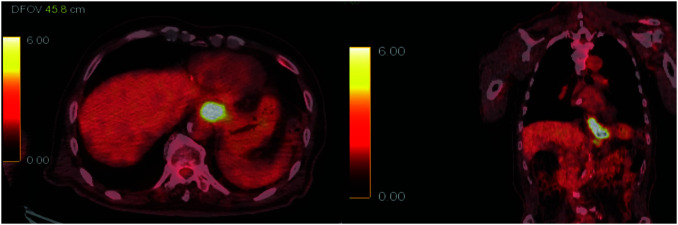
Fluorine-18 FDG PET/CT fusion image (axial slice). Intense FDG uptake is seen in the distal esophageal/EGJ tumor (SUV ~12.6). EGJ, esophagogastric junction; FDG, fluorodeoxyglucose; SUV, standardized uptake value
